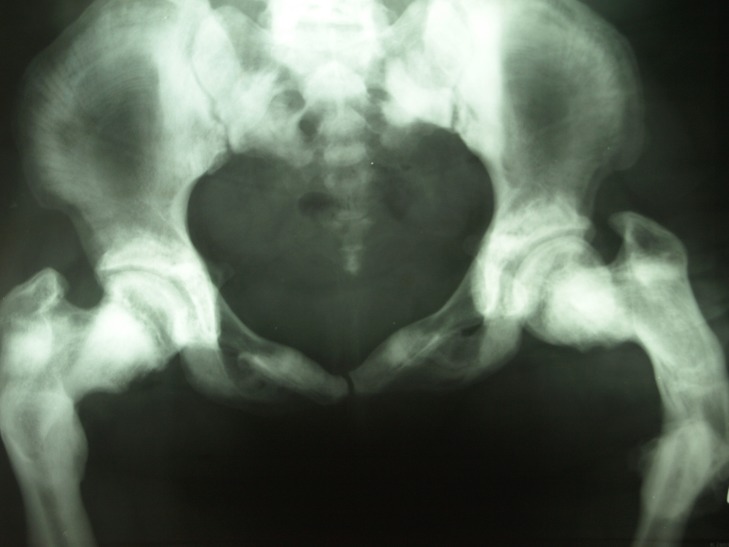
Radiograph of pelvic bones showing fracture
